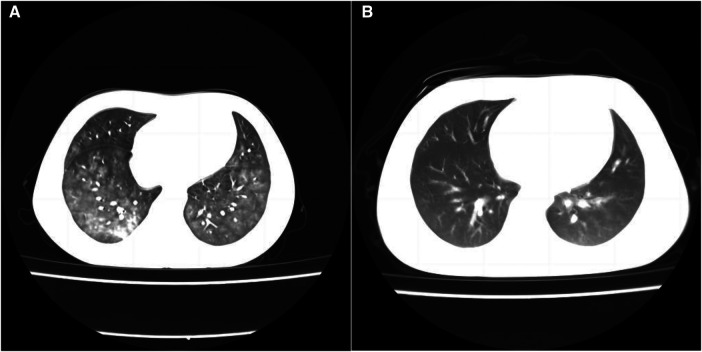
Chest CT scanning of the patient. CT lung window on admission showed diffuse small ground-glass opacities in both lungs with patchy opacities in the right lower lobe, and diffuse alveolar hemorrhage was considered (A). The CT lung window on the 11th day after admission showed a little inflammation in both lungs, and the lesions in both lungs were significantly reduced and faded (B).
